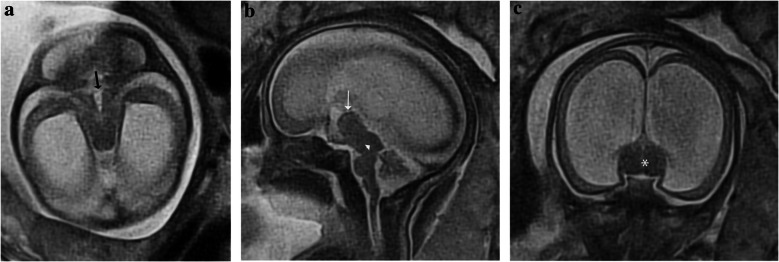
Diencephalic-mesencephalic junction dysplasia and L1CAM mutation variant of unknown significance. Fetal MRI was performed at 22 weeks for further evaluation of severe ventriculomegaly identified sonographically. Axial (a) T2 single-shot fast-spin echo (SSFSE) image through the brainstem shows abnormal contour with deep ventral cleft (*black arrow*). Sagittal (b) SSFSE image demonstrates an enlarged and ventrally positioned interthalamic adhesion (*white arrow*), nonvisualization of the cerebral aqueduct (*white arrowhead*), and normal size and shape of the fourth ventricle. Coronal (c) SSFSE image shows diencephalosynapsis (*asterisk*) and severe ventriculomegaly
